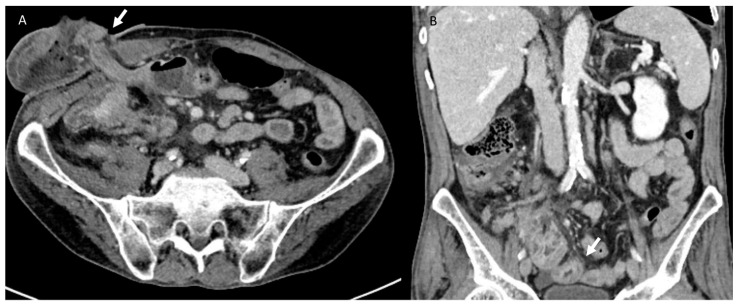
CT assessment of stomal hernia. In (A) (axial plane), the arrow shows parastomal fat accumulation at the stoma site. In (B) (MPR coronal plane), the arrow shows intestinal wall edema.
